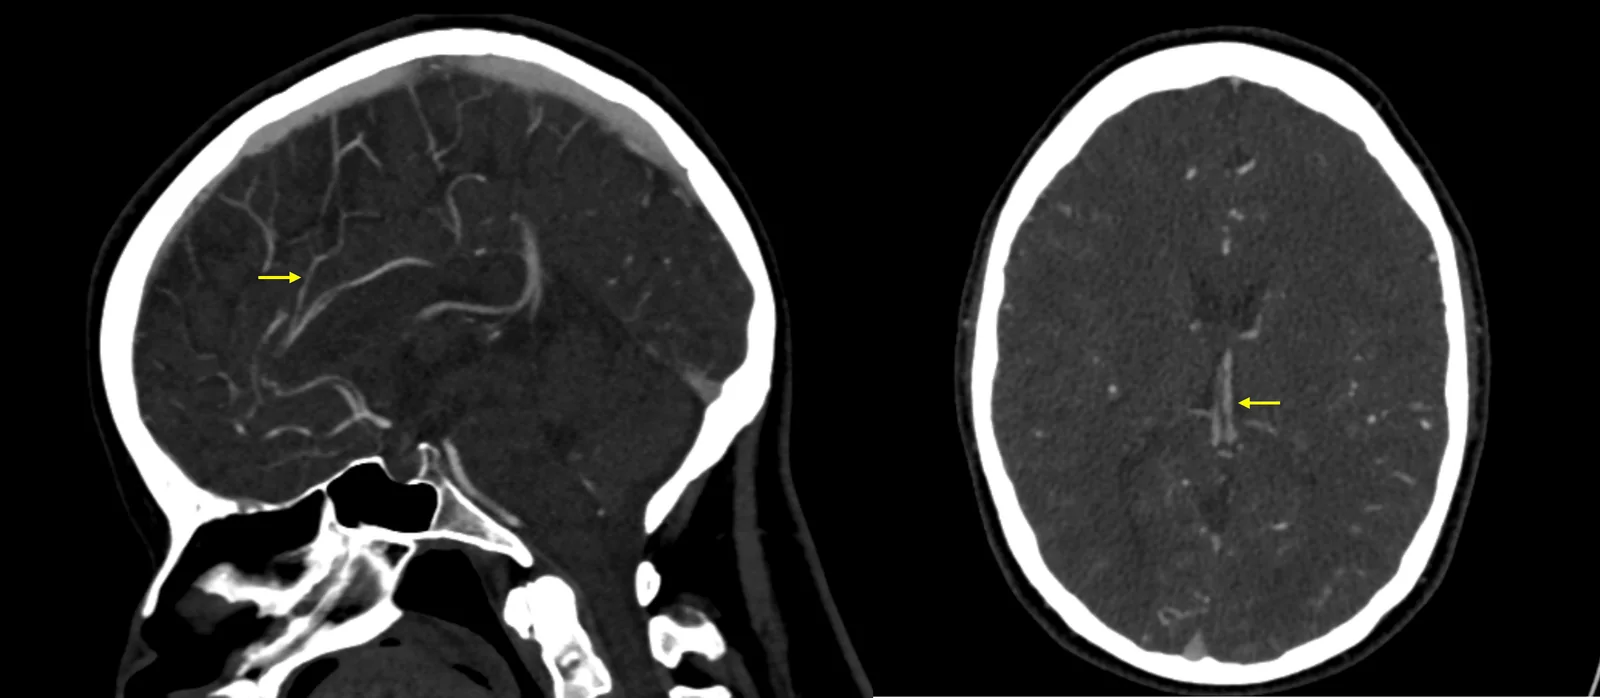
Head CT images showing obliterative irregularities on cortical arteries and the pericallosal arteries (sagittal and axial planes).
